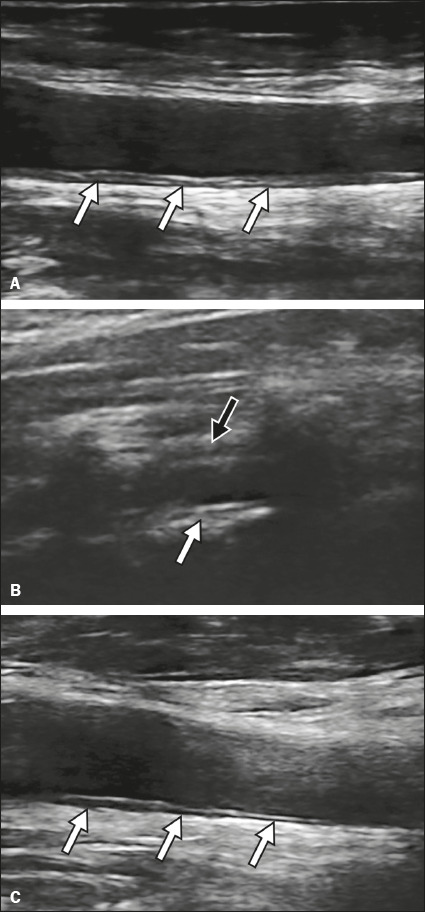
A 50-year-old man with COVID-19. Grayscale ultrasound images showing a
